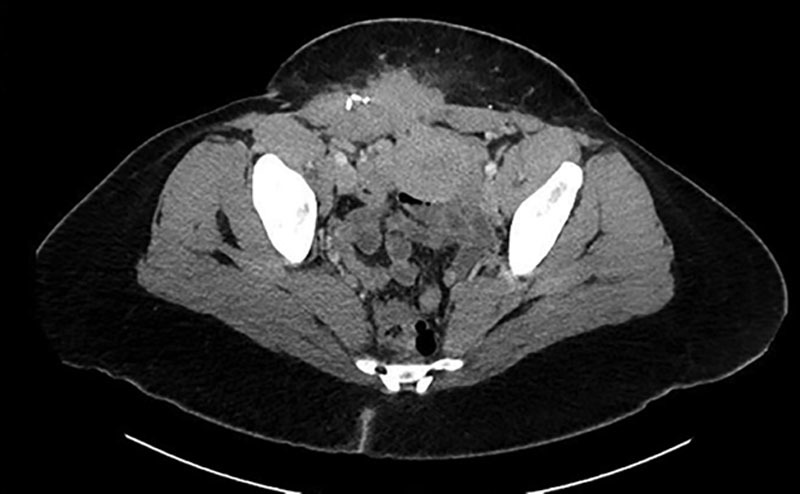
Computed tomography image showing infiltrating mass within abdominal wall.
